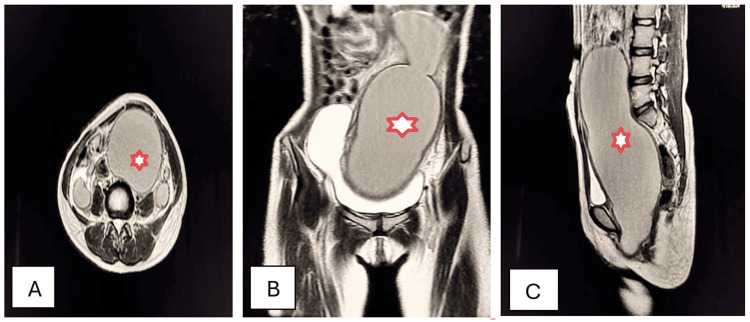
Hematometra and hematocolpos due to imperforate hymen (stars). Axial (A), coronal (B), and sagittal (C) T2W MRI imaging shows a huge enlargement of the uterus with thinning of the myometrial and cervical walls due to hypointense endometrial and endocervical collection (with the shading sign due to recurrent hemorrhage), consistent with hematometra and hematocolpos caused by an imperforate hymen. T2W: T2-weighted
